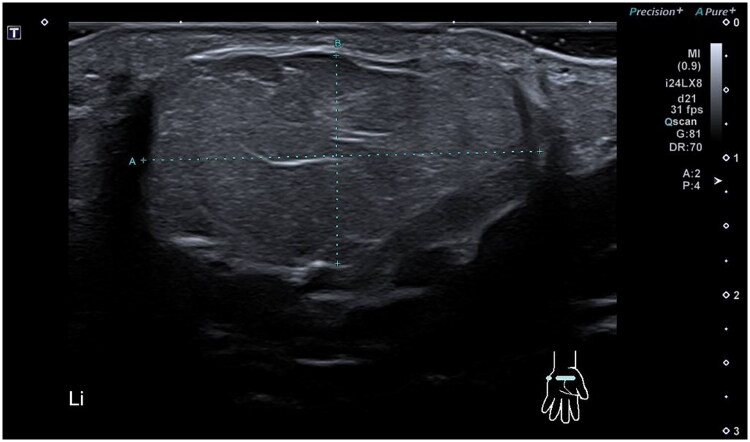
Ultrasound cross-section of the lipoma.
